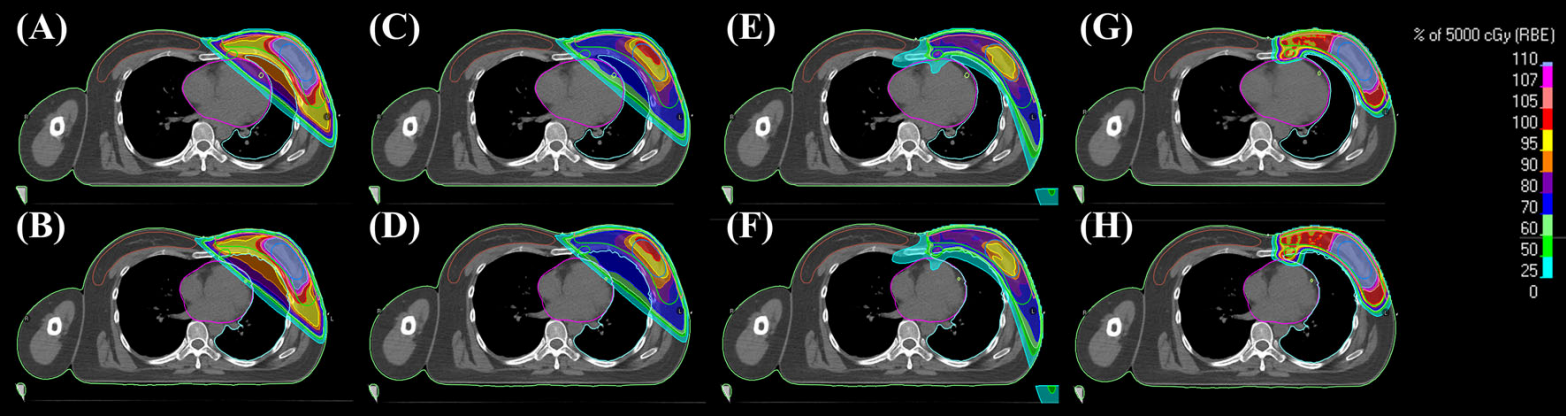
Representative 2D dose distributions in a patient. (A) 3DCRT-FB-CF, (B) 3DCRT-CPAP-CF, (C) 3DCRT-FB-HF, (D) 3DCRT-CPAP-HF, (E) VMAT-FB-HF, (F) VMAT-CPAP-HF, (G) PBT-FB-CF, and (H) PBT-CPAP-CF on the same level cut of CT images. 3DCRT, three-dimensional conformal radiotherapy; VMAT, volumetric modulated arc therapy; PBT, proton beam therapy; CPAP, continuous positive airway pressure; CF, conventional fractionation; HF, hypofractionation.
